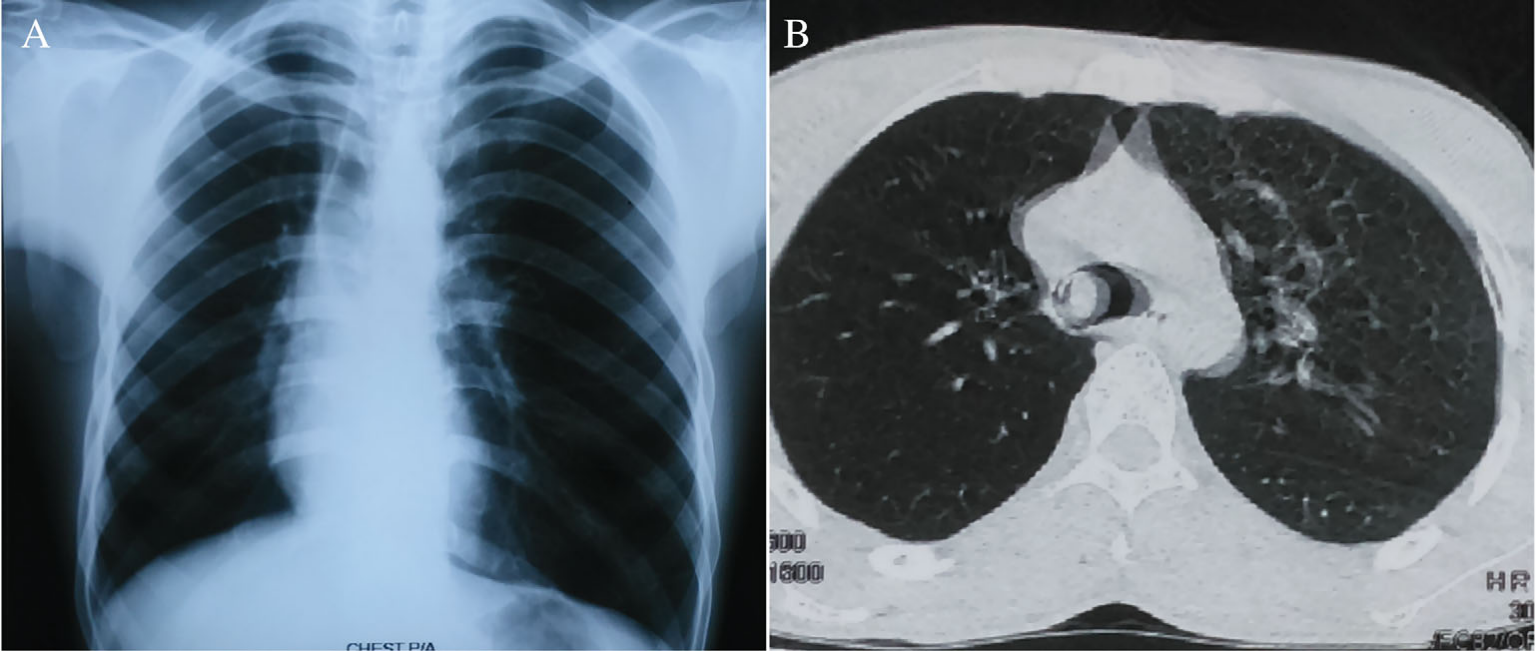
Chest X‐ray P/A view showing the right upper lobe collapse (A), and a polypoid mass at the level of carina on computed tomography scan of the chest (B).
